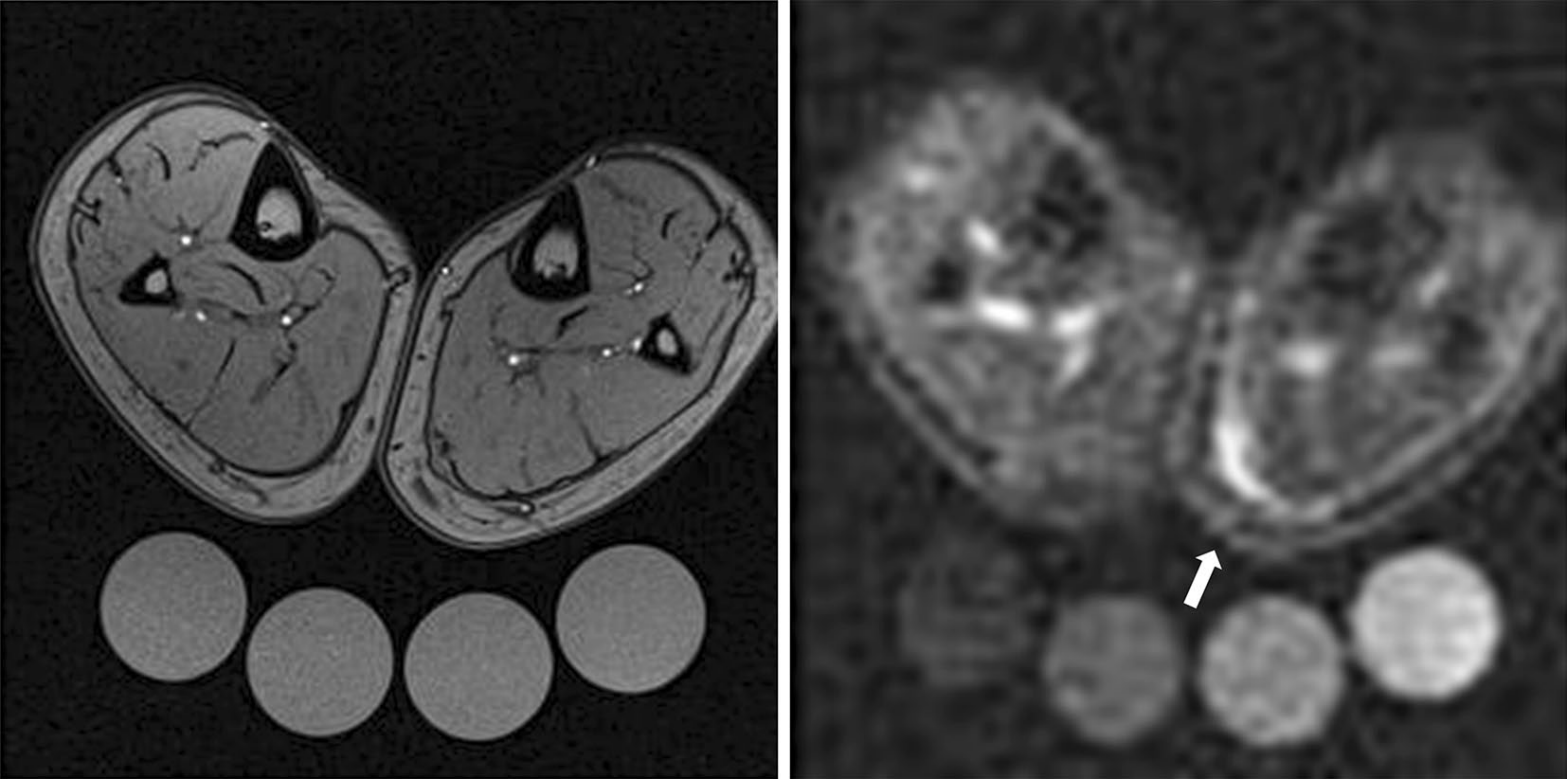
^1^H-MR imaging [T1-weighted fast-low-angle-shot (FLASH)-sequence, *left*] and ^23^Na-MR imaging (*right*) of both lower legs 2 weeks after injury. The area of hyperintense Na^+^ rich tissue was reduced, but could still be clearly visualized at the level of the left medial gastrocnemius muscle (*arrow*, Na^+^ concentration compared to the corresponding soft tissue of the contralateral non-affected leg: 37.5 vs. 18.5 mmol/l). Muscle function of the leg was completely restored by this point
